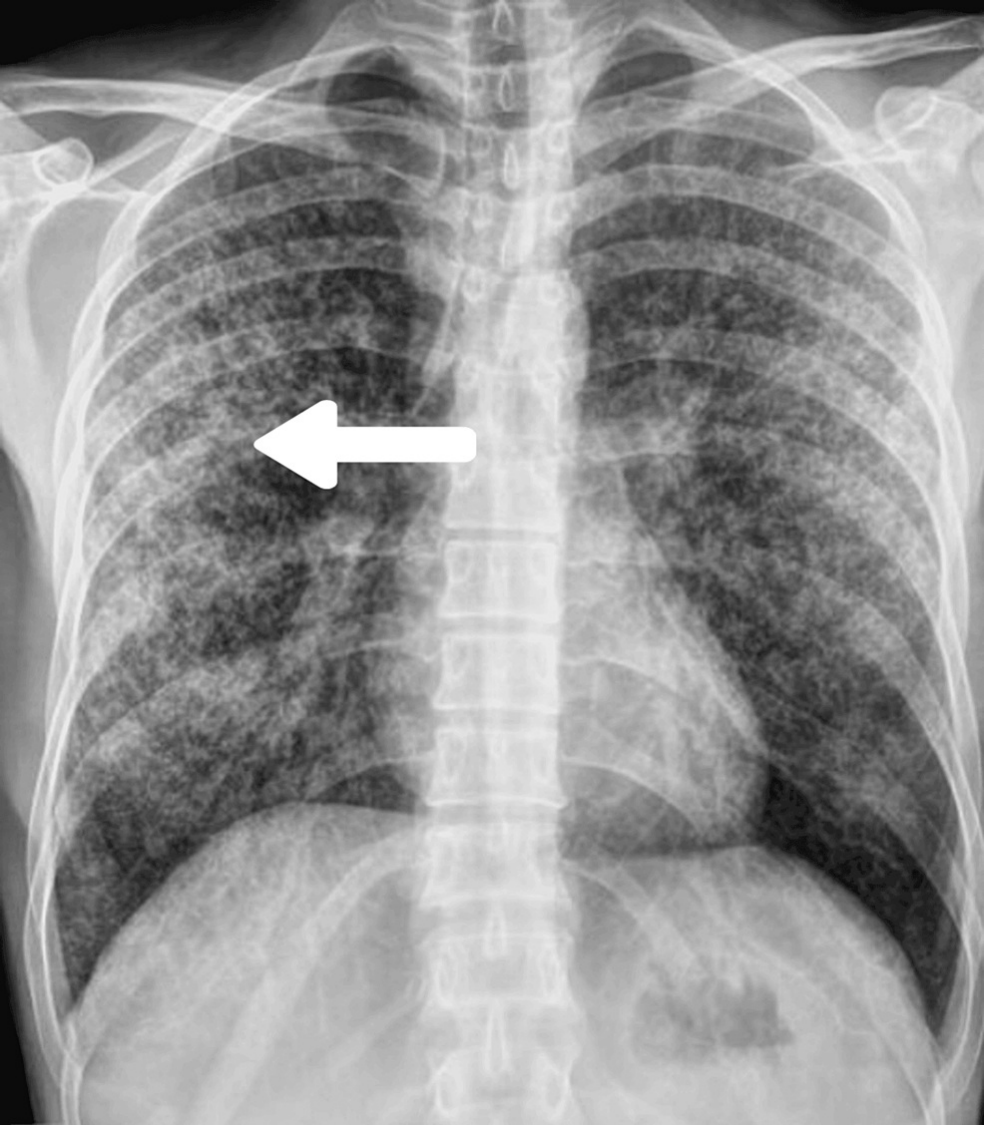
Chest radiograph showing miliary pattern (white arrow) which is suggestive of tuberculosis
